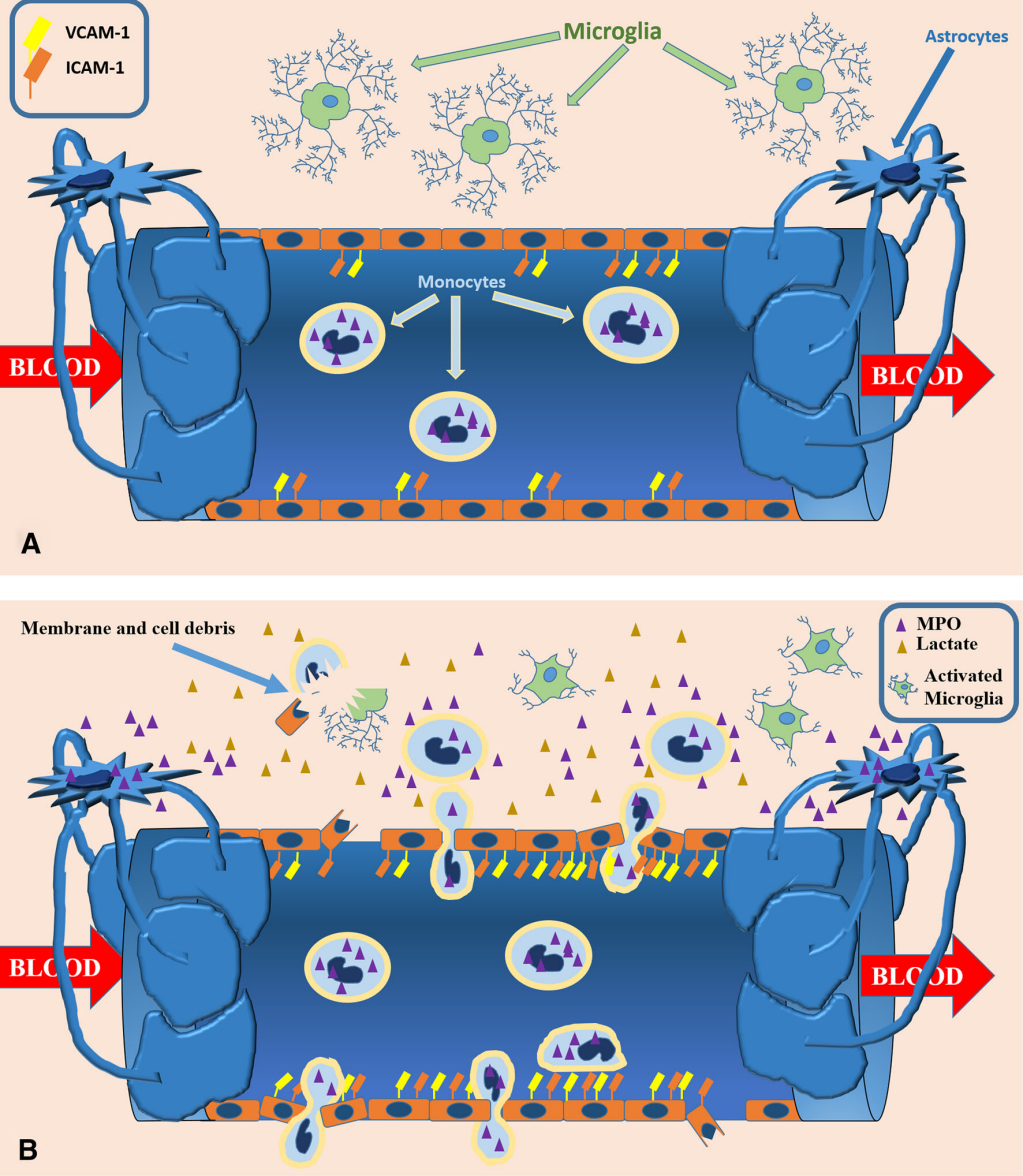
Main neuroinflammatory mechanisms probed by MRI. Modifications of the neurovascular unit include alterations of the BBB permeability, associated with overexpression of adhesion molecules, which induce blood-borne monocytes to arrest and crawl along the endothelium, crossing it by diapedesis along loosened intercellular junctions. Machrophage and microglia activation results in increased MPO activity and lactate accumulation in the interstitium, along with the presence of cellular debris resulting from cell damage due to oxidative and excitotoxic phenomena
